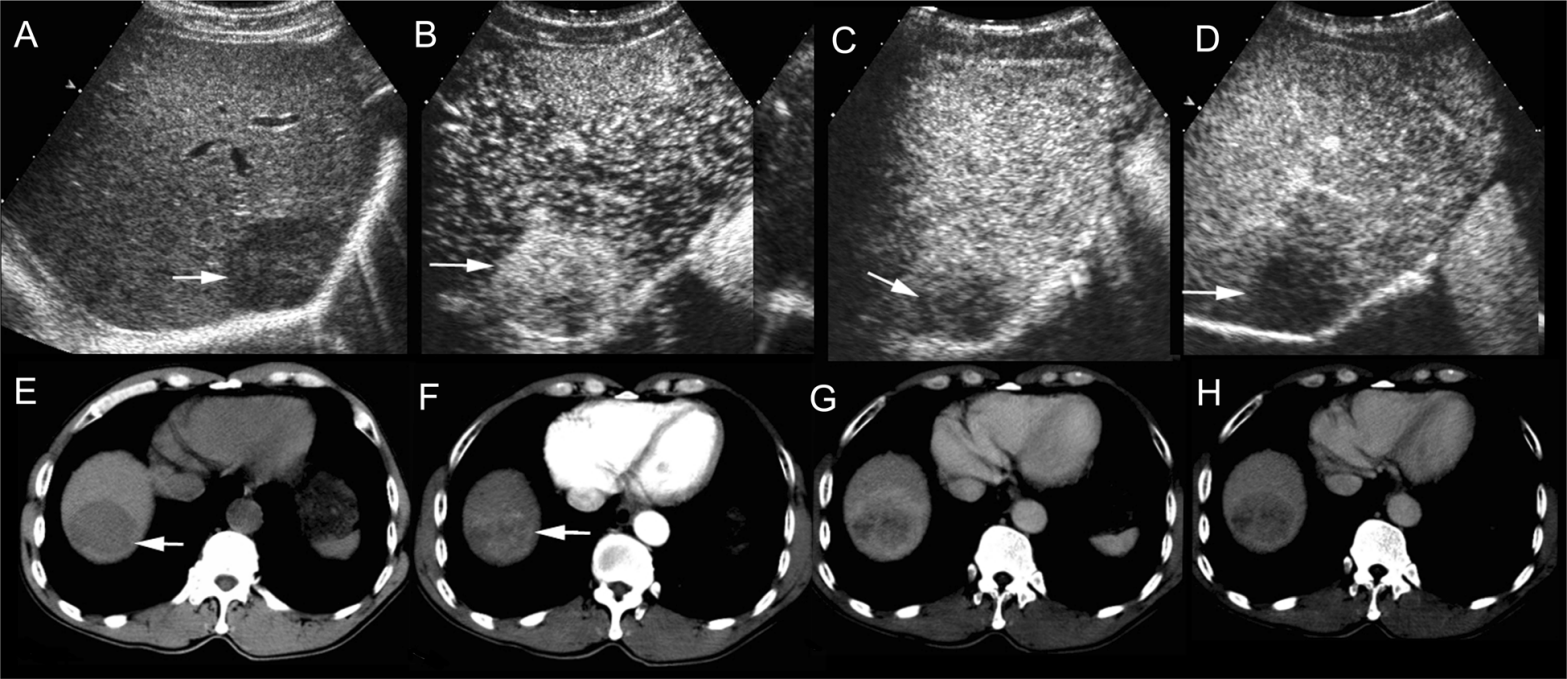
cHCC-CC in a patient with chronic hepatitis B. Simultaneous elevation of AFP (718 ng/ml) and CA19-9 (45.0U/ml) was detected in the patient. Unenhanced ultrasound shows a hypoechoic mass of 4.6 cm in right lobe of the liver (a, arrow). The mass displays heterogeneous hyperenhancement in the arterial phase (b, 26 s after contrast agent injection) followed by quick (c, 58 s after injection) and marked washout (d, 102 s after injection) in the portal phase on CEUS, resembling the enhancement pattern of intrahepatic cholangiocarcinoma. Unenhanced CT scan reveals a hypodense mass in right lobe of the liver (e, arrow). The mass displays heterogeneous hyperenhancemen in the arterial phase (f, arrow) followed by washout (g) in the portal phase and the late phase (h) on contrast-enhanced CT, resembling the enhancement pattern of HCC
